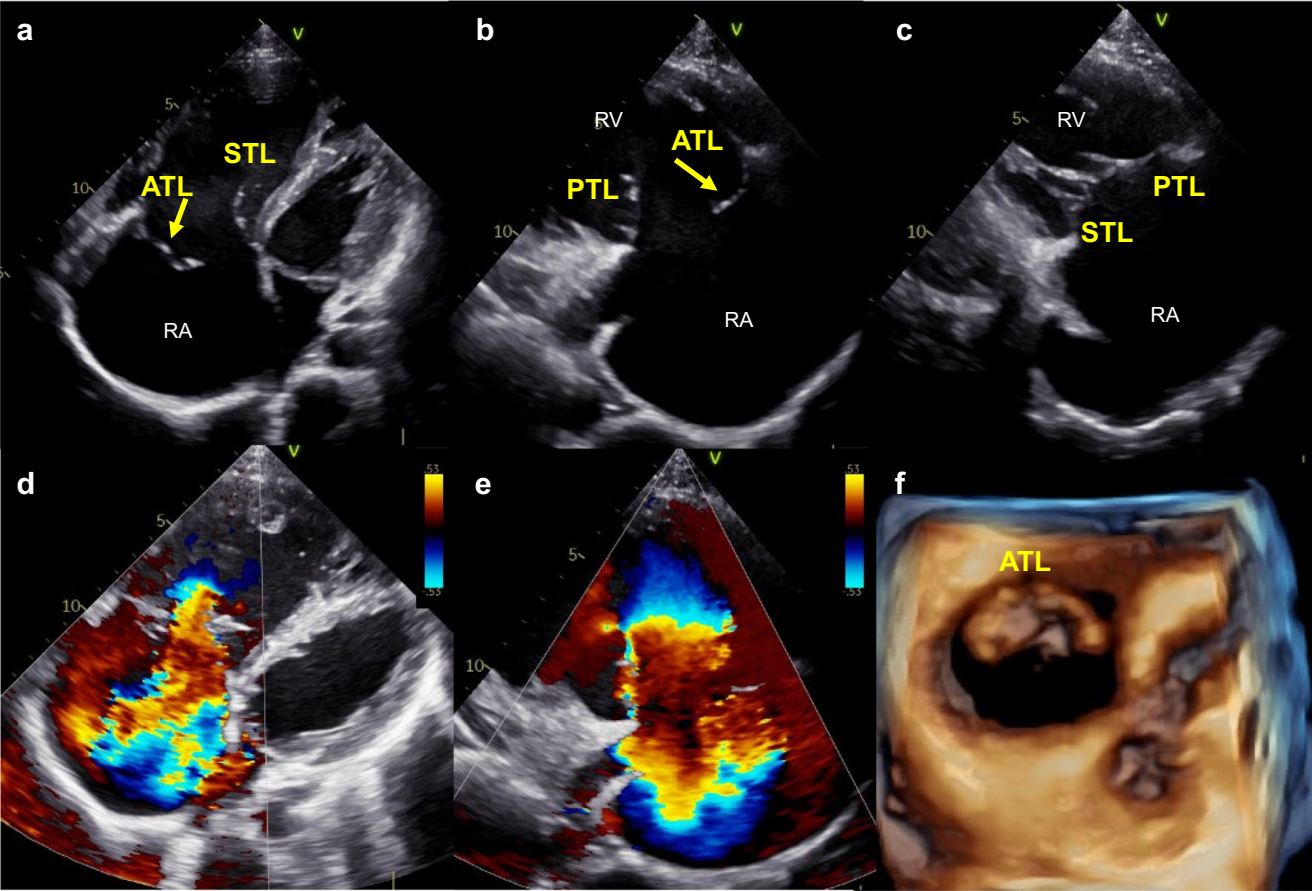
a Two-dimensional transthoracic apical four-chamber view showed prolapse of the ATL (arrow) and enlargement of the RA and right ventricle. (b, c) Two-dimensional transthoracic parasternal right ventricular inflow view showed prolapse of the ATL (arrow) and good coaptation of the STL and PTL. (d, e) Color flow Doppler showed severe TR. f Three-dimensional view from the right atrial aspect showed prolapse of the ATL. RA, right atrium; ATL, anterior tricuspid leaflet; STL, septal tricuspid leaflet; TR, tricuspid regurgitation; PTL, posterior tricuspid leaflet
